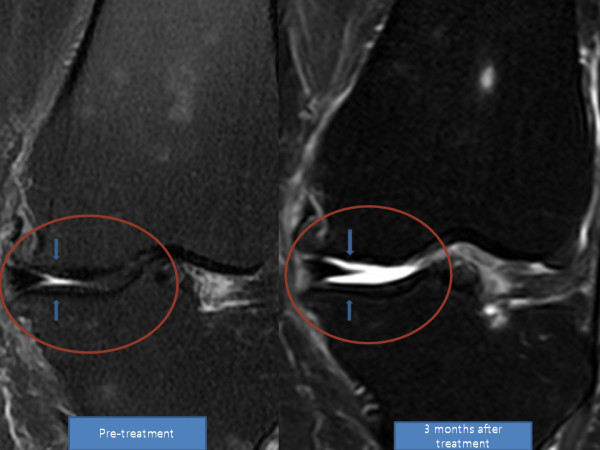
MRI coronal T2 view of the knee. The anterior medial meniscus has increased in height.
